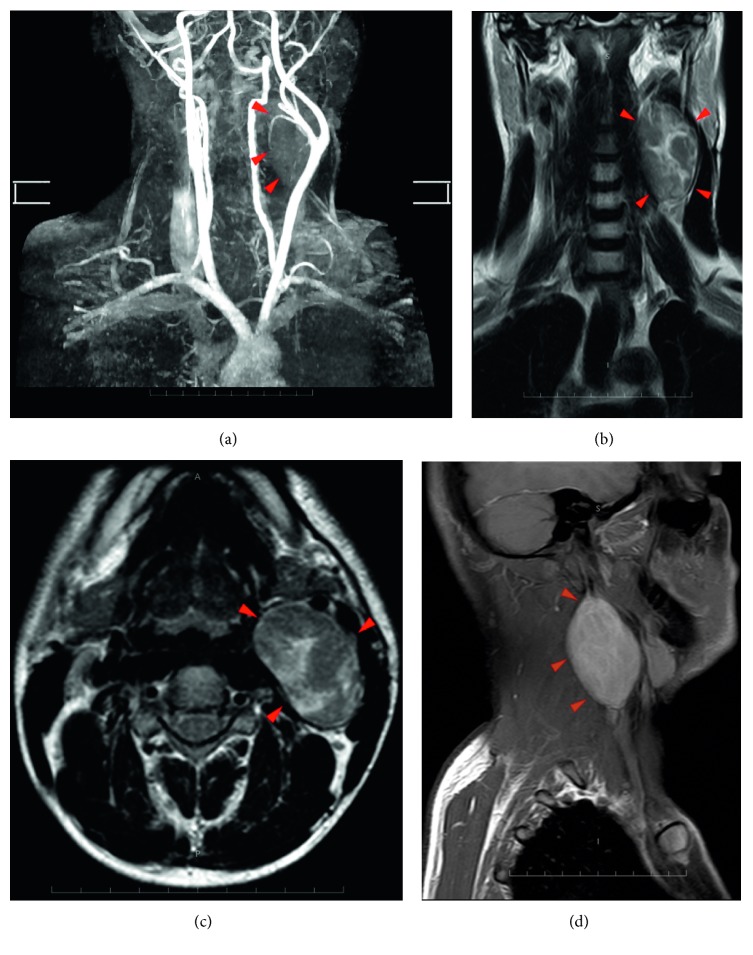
(a) MRI angiography showing splaying of the carotids by the tumor. (b) T2-weighted coronal cut. (c) T2-weighted axial cut. (d) Postcontrast T1 sagittal cut.
